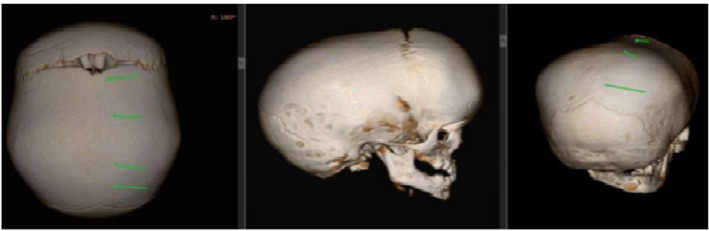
CT 3D reconstruction of skull of the male sibling.
